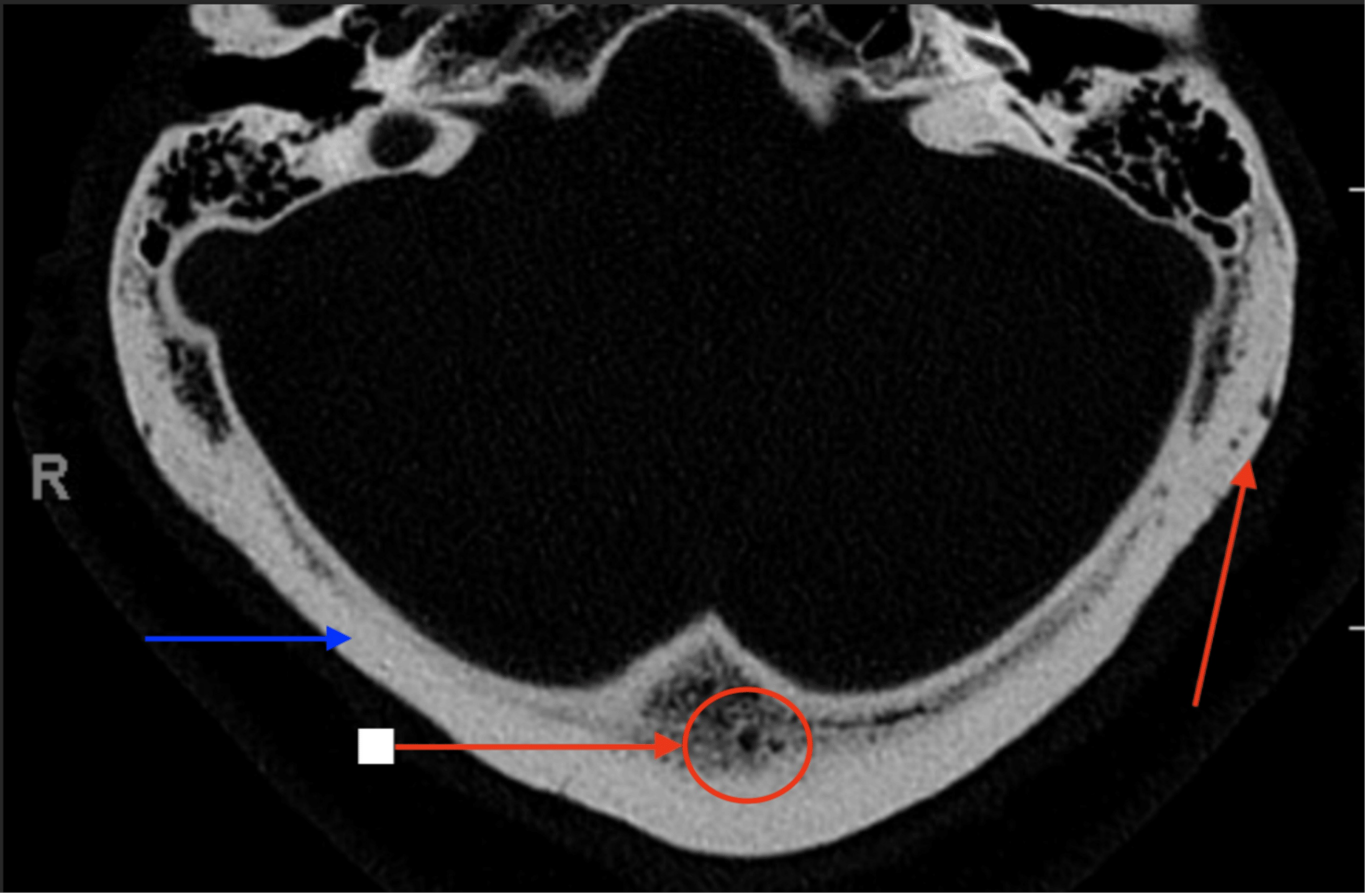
Axial CT of the calvarium showing lytic lesions
